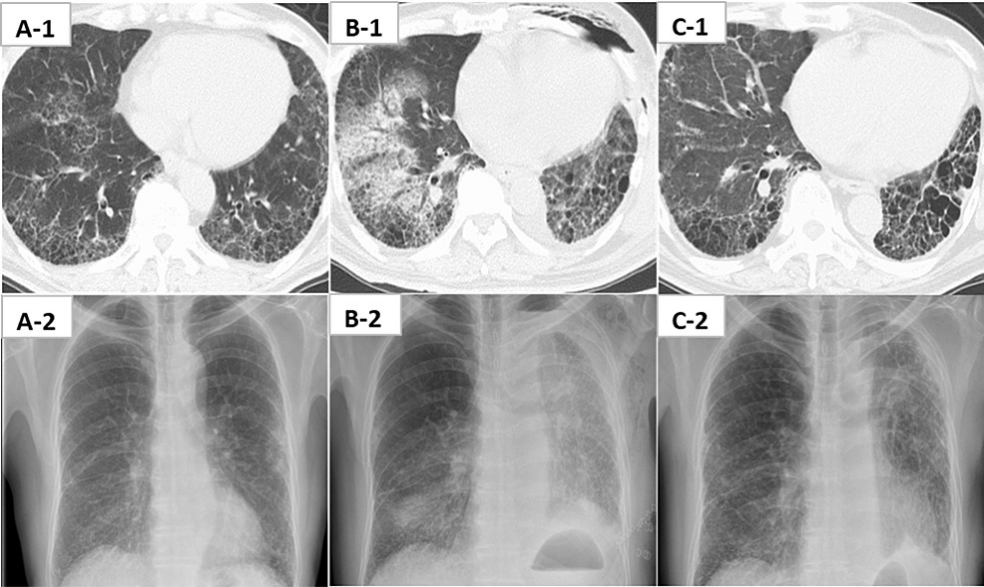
Demonstrable images of computed tomography (1) and chest x-ray (2). (A) Before surgery. (B) During acute exacerbation (POD6). (C) After 18 months. POD; postoperative day
